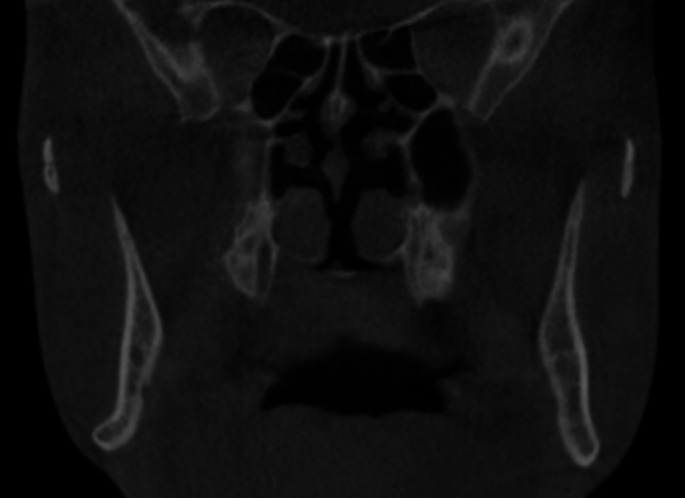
A CBCT coronal cut showing the thin mandibular rami, rolling out of the inferior mandibular border, and the IAN proximity to the buccal cortex
